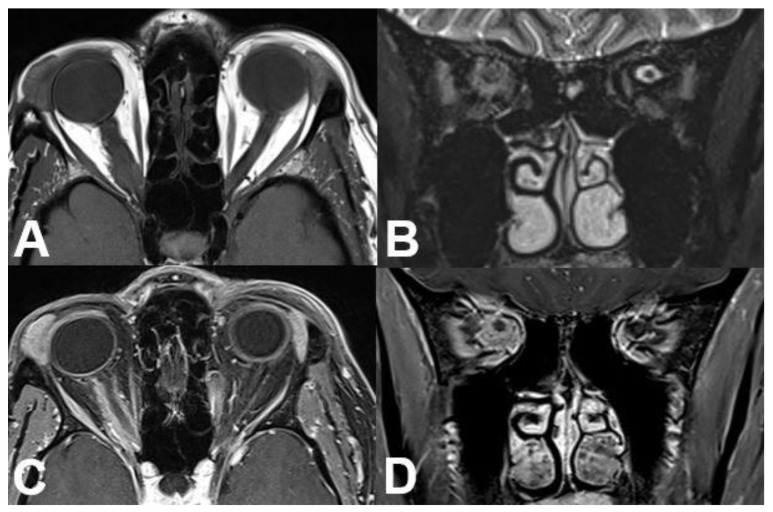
Optic nerve sheath meningioma (ONSM).* MRI of a 43-year-old female patient with a well-defined mass around the optic nerve with T1-hypointense (A) and T2-hyperintense signal (B). After contrast administration (C,D), a homogenous enhancement is seen with sparing of the optic nerve (“tram track sign”).
